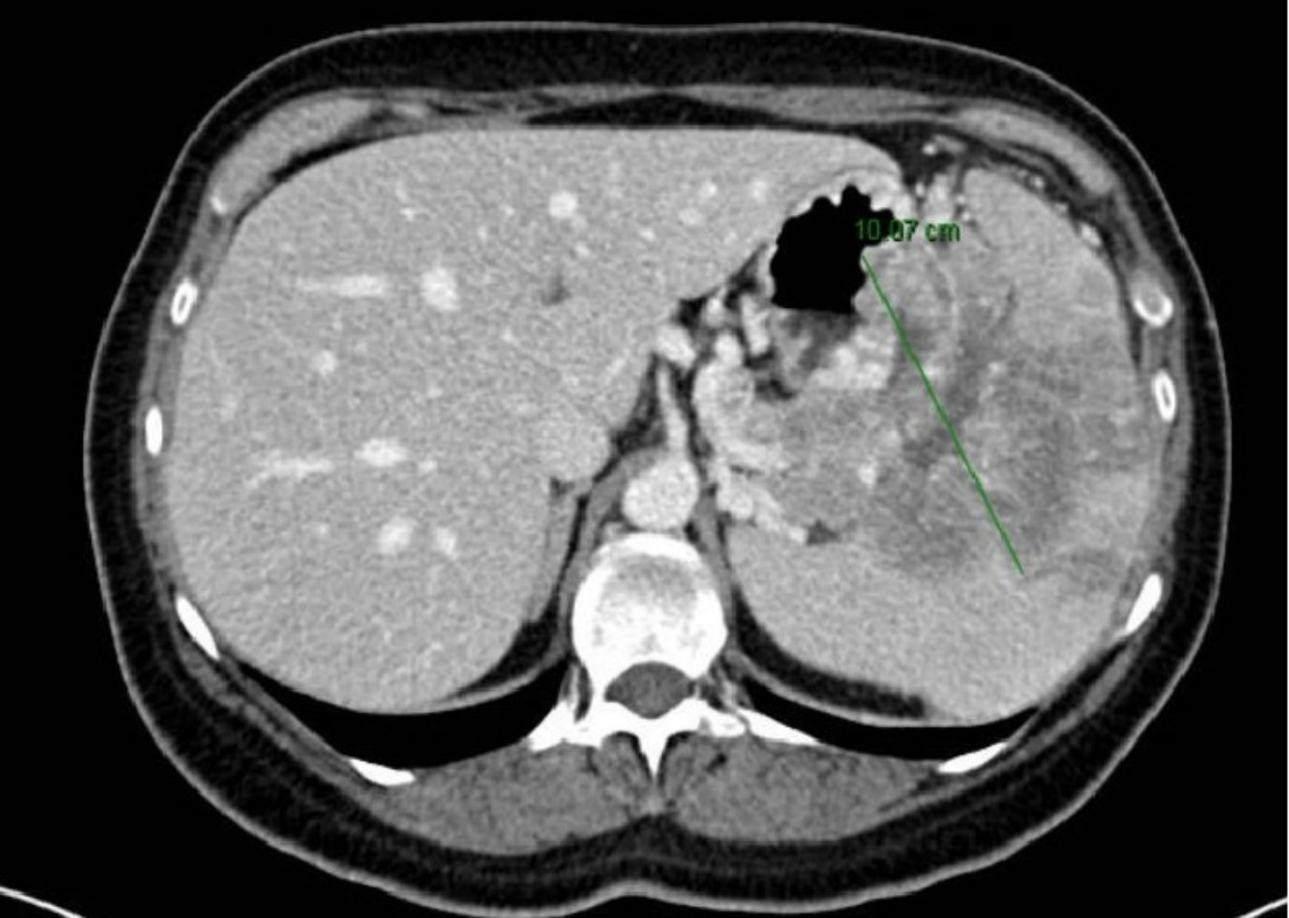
Computed tomography (CT) scan of the abdomen showing a big mass in the body and tail of the pancreas CT scan examination showed an 8 x 10 cm mass in the body and part of the tail of the pancreas with hypodense non-enhancing areas suggestive of necrosis or cystic change.
